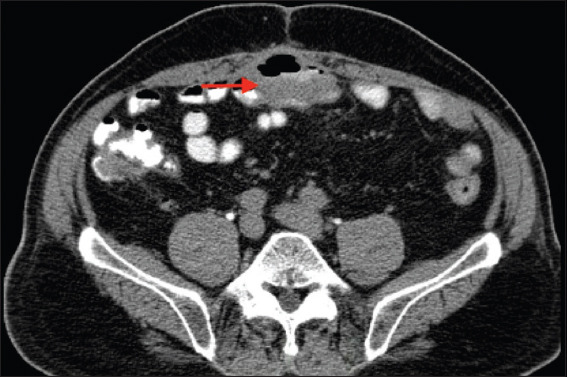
Abdominopelvic computed tomography scan displaying an anterior abscess (red arrow) at the site of a previous hernia repair with synthetic mesh.
